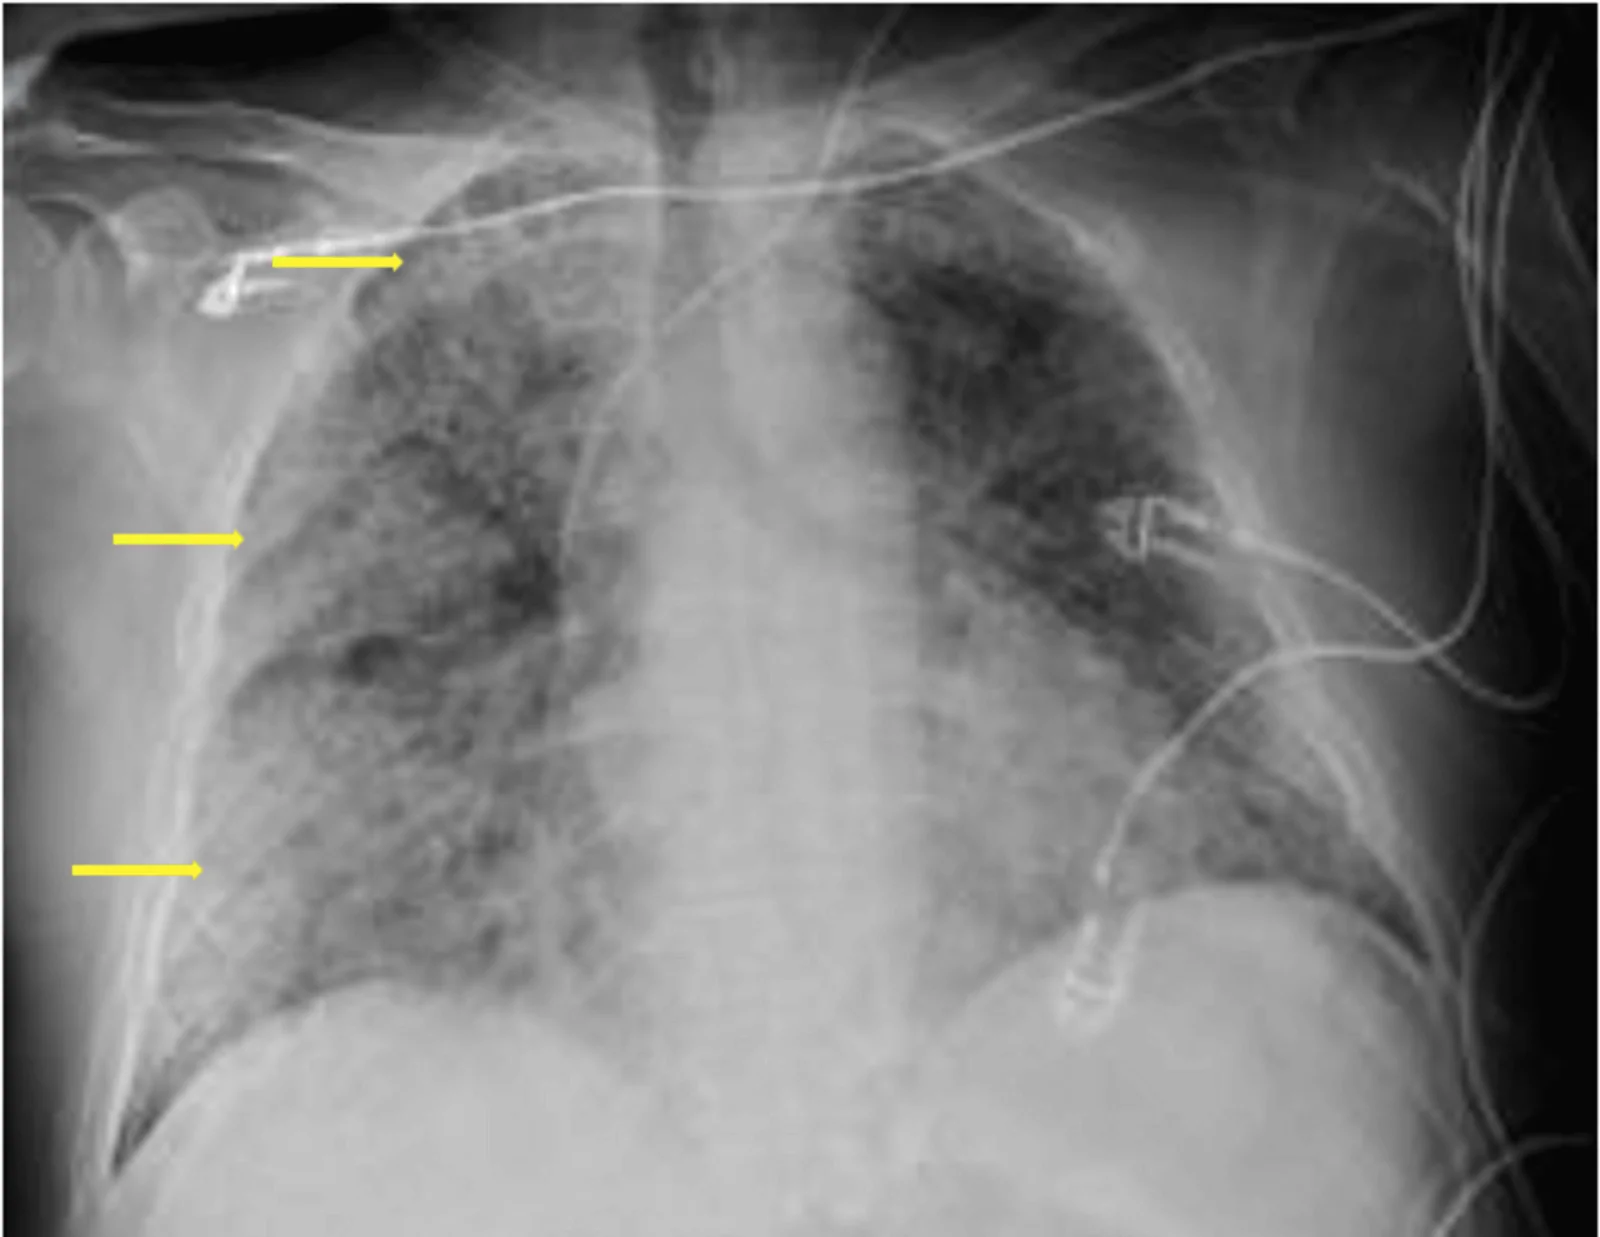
Chest X-ray, anteroposterior (AP) view, showing acute respiratory distress syndrome (ARDS). Diffuse alveolar and interstitial infiltrates distributed peripherally in both lungs, more pronounced on the right side, as indicated by the yellow arrows.
